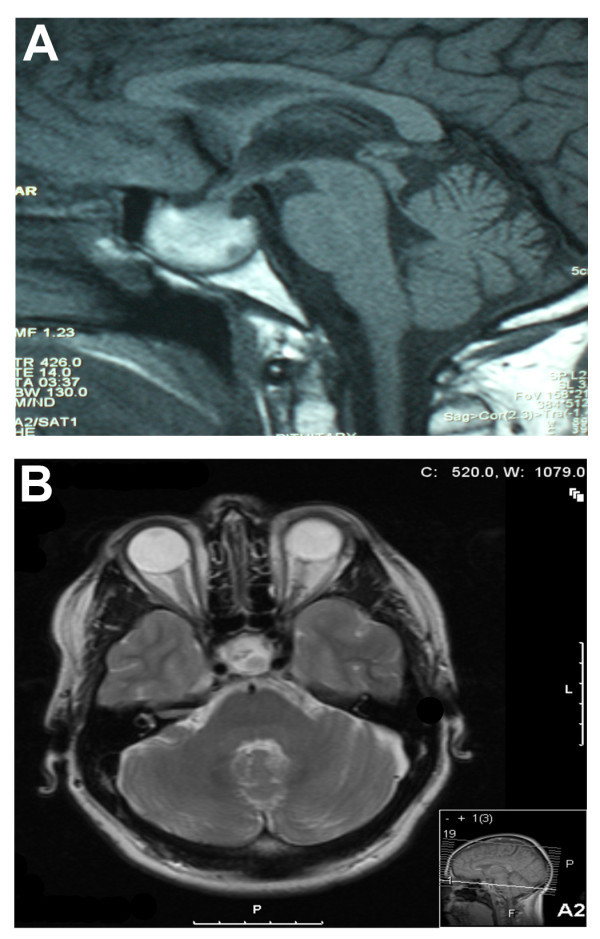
MR imaging of pituitary apoplexy. a A sagittal T1 image of the pituitary gland. The enlarged sella containing an abnormal soft tissue with increased signal intensity is suggestive of haemorrhage. b A transverse T1 image of the pituitary gland 2 months following presentation. The sella is expanded and contains abnormal soft tissue that is of low T1 signal. A small area of high T1 signal in the superior aspect of this is taken to represent normal posterior pituitary signal. There are no features of haemorrhage on this later scan.
